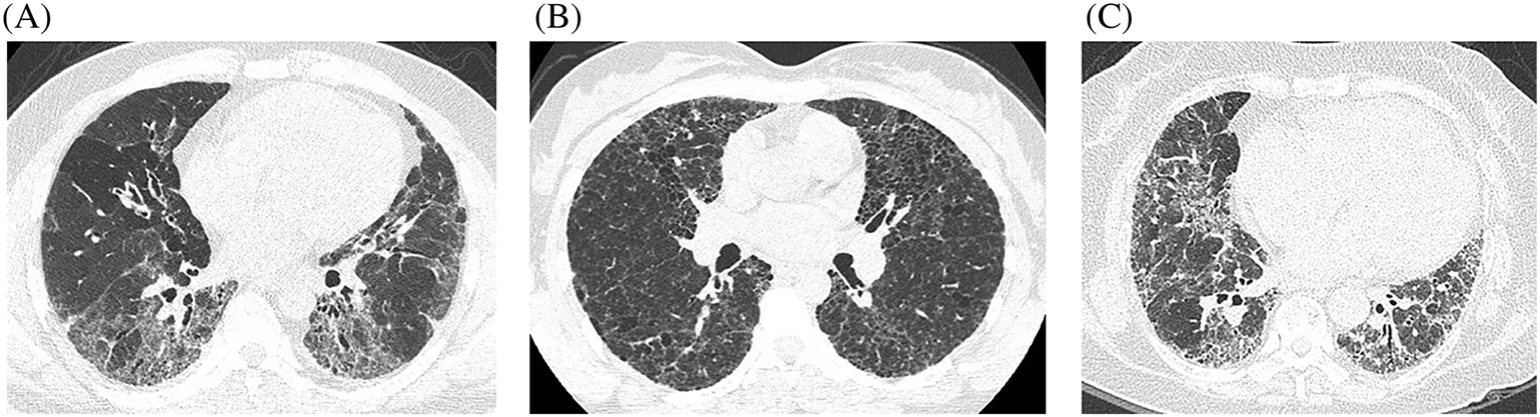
HRCT results in patients with fibrotic HP. (A) A male patient with fibrotic HP. HRCT showed reticulation, traction bronchiectasis, and mosaic attenuation. (B) A female patient with fibrotic HP. HRCT showed reticulation and honeycombing. (C) A female patient with chronic fibrotic HP. HRCT showed reticulation and a “three density pattern” (headcheese sign). HRCT, high‐resolution computed tomography; HP, hypersensitivity pneumonitis
